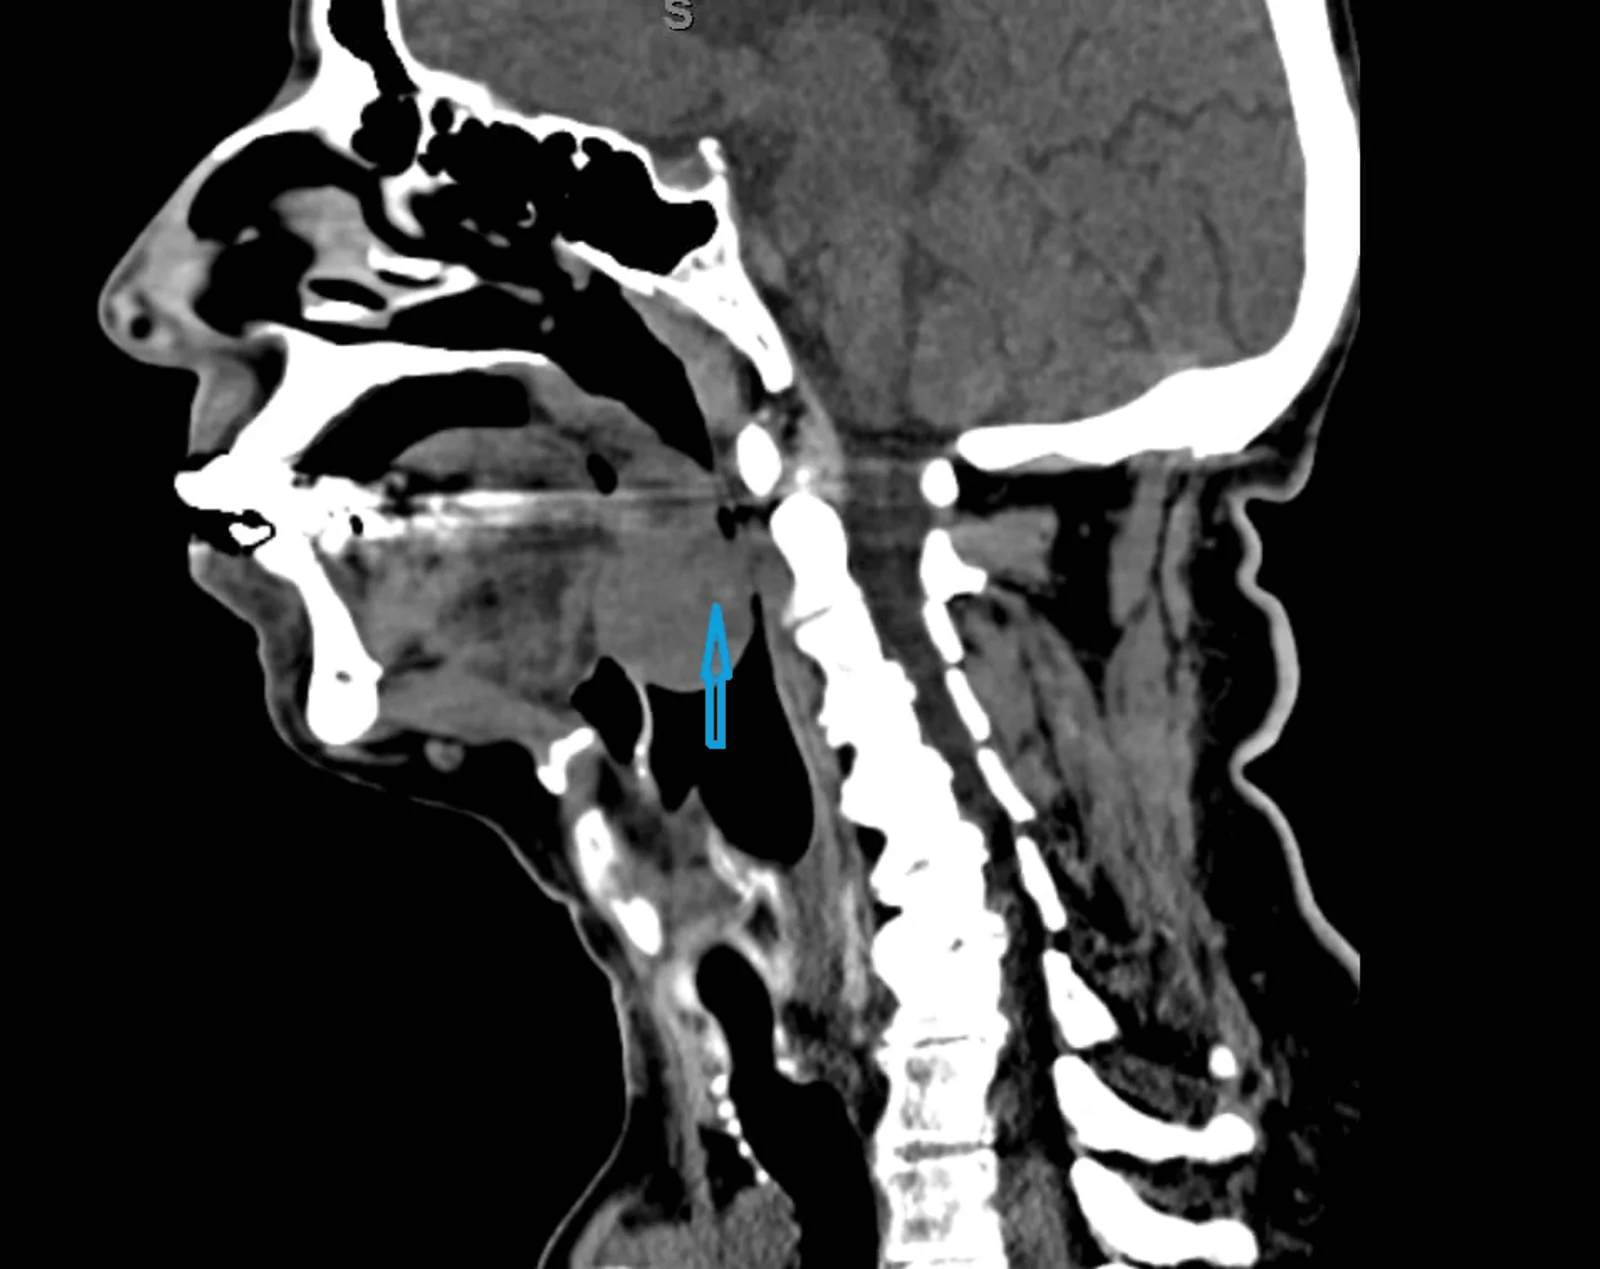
CT image showing homogeneous and intensely enhancing, lobulated, exophytic centered within the right tonsillar fossa (blue arrow). The lesion abutted the right base of the tongue, protruded into the oropharyngeal airway, and extended laterally toward the pterygoid musculature (blue arrow).
